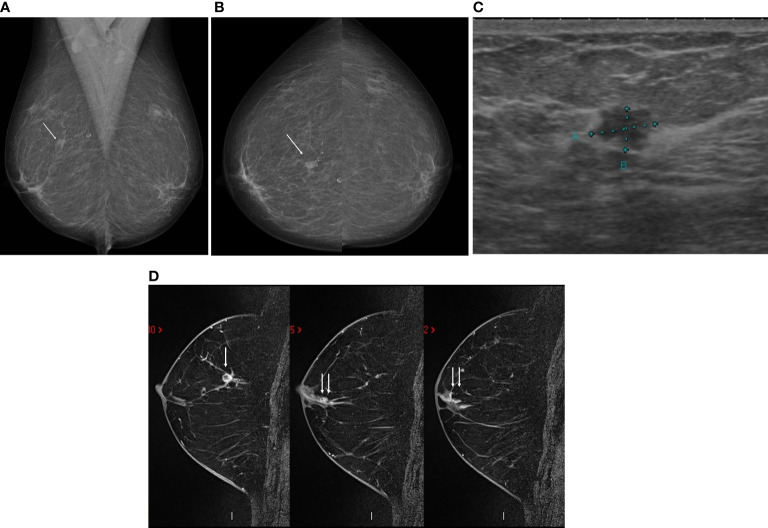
A 58-year-old asymptomatic patient underwent mammography in the mediolateral oblique (A) and cranio-caudal (B) views, which showed an irregular nodule, low density and indistinct margins in the middle third of the upper quadrants of the right breast (arrow). Ultrasound showed an irregular, hypoechoic nodule, with non-circumscribed margins, parallel to the skin, with no sound change in this topography (C), with percutaneous biopsy demonstrating invasive ductal carcinoma, moderate grade, negative for estrogen and progesterone receptors (Luminal A). Magnetic resonance imaging for staging confirmed the nodule at the junction of the upper quadrants of the right breast (arrow), in addition to demonstrating non-nodular enhancement extending from the nodule to the papilla (double arrow), confirmed as ductal carcinoma *in situ*
